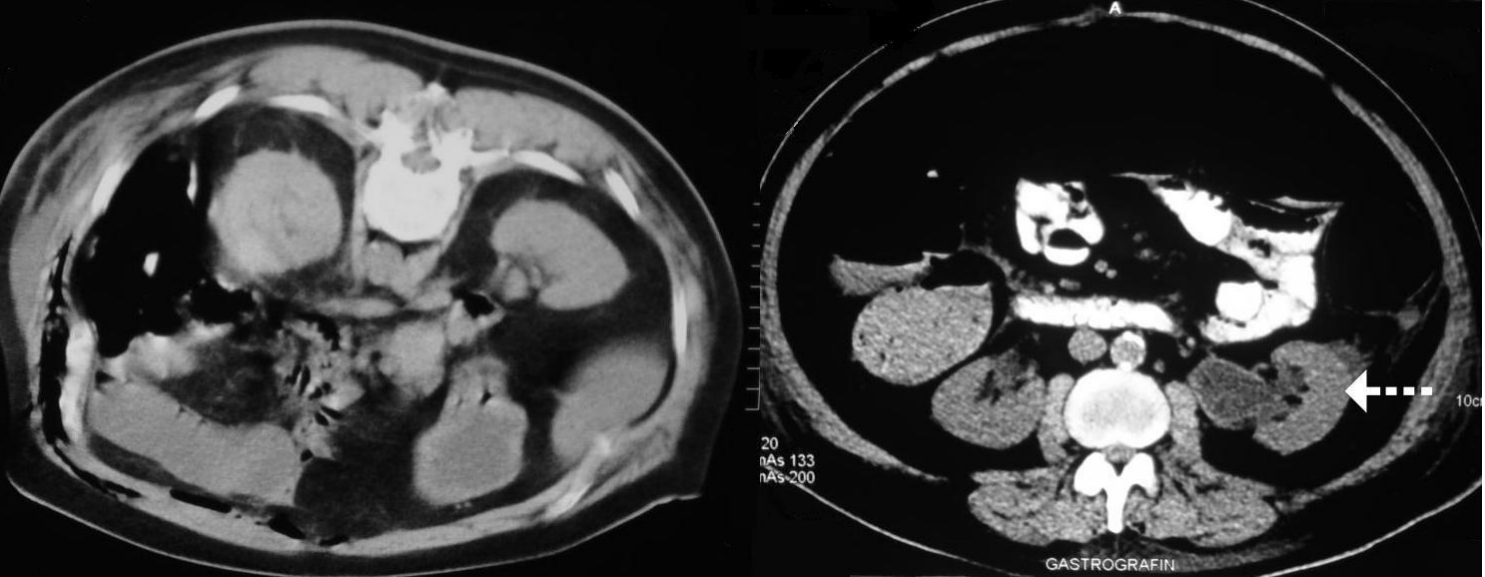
CT scan of the abdomen revealed extensive collections of gaseous fluid mainly in the left retroperitoneum extending along the paracolic gutter and to the middle. The air in the dilated left renal calyces gives the only clue to the diagnosis (arrow).
